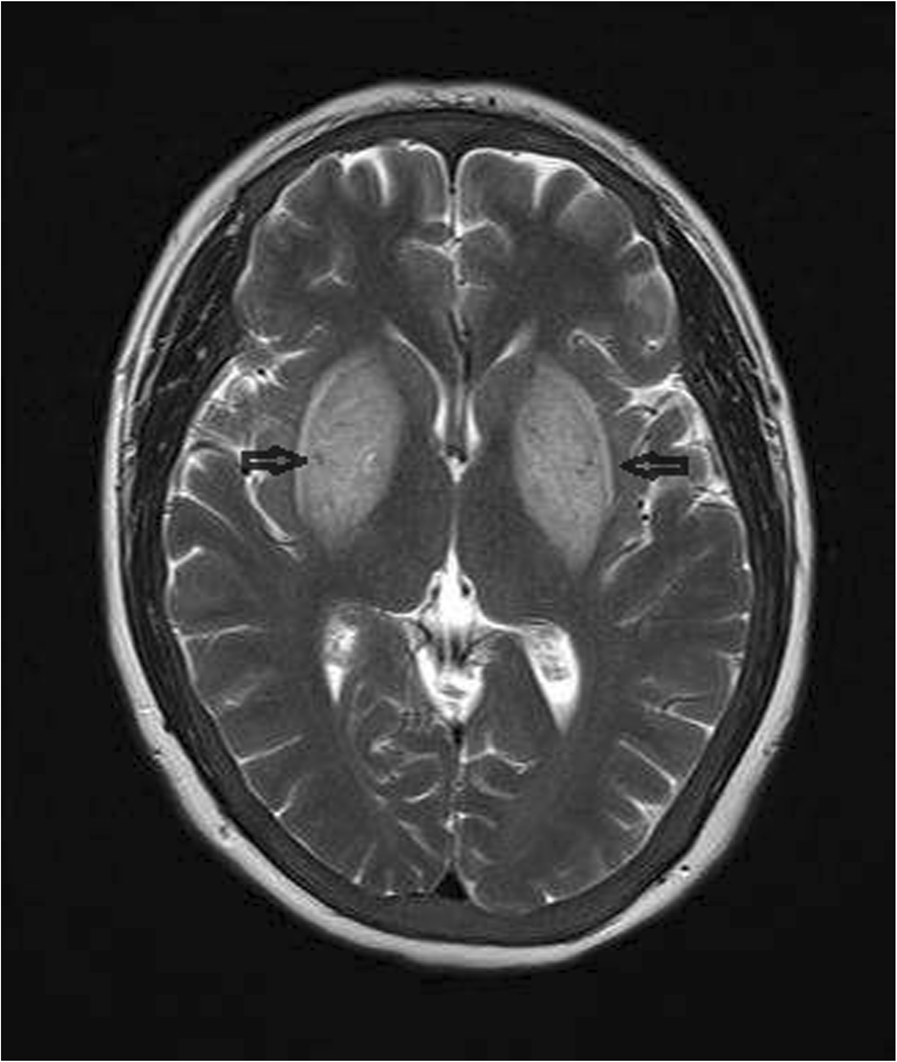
Brain MRI performed upon admission. T2WI showed symmetrical hyperintense non-hemorrhagic lesions in the entire bilateral basal ganglia (arrows), and corona radiata lesions showing mild diffusion restriction
